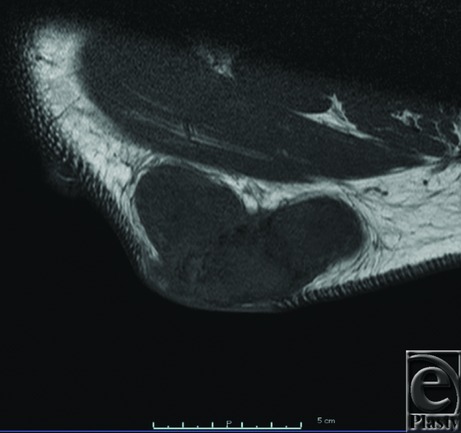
Signal intensity of the tumor on the right shoulder on magnetic resonance images. On this T1-weighted image, the tumor shows iso/low signal intensity.
